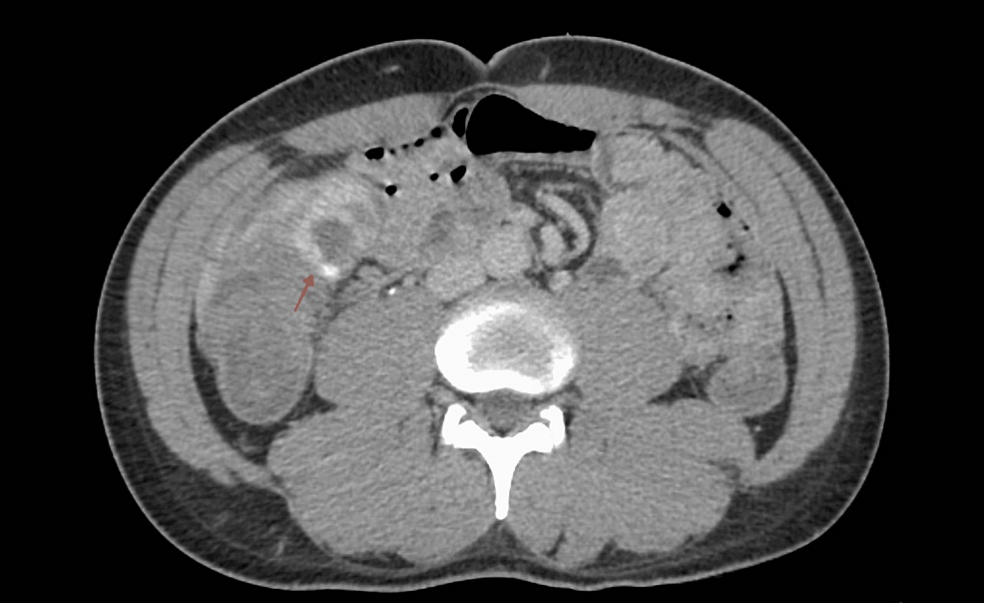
Computed tomography angiography acute gastrointestinal bleeding protocol revealing extravasation in the cecum and proximal ascending colon (red arrow).
